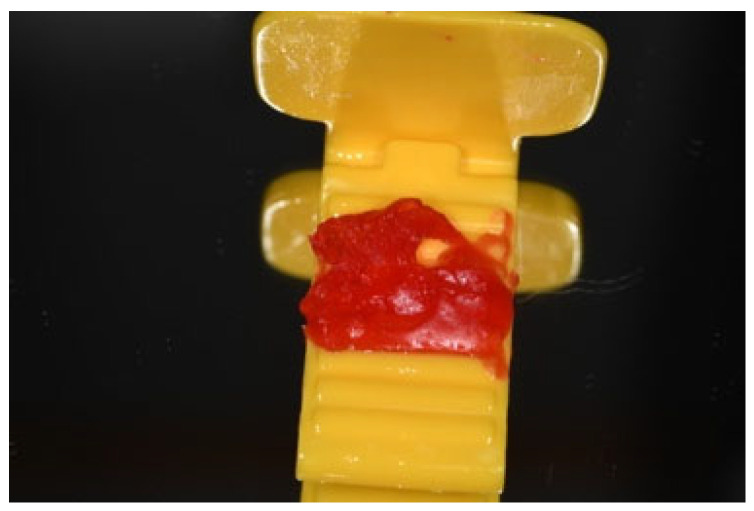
Customized film holder used for each time-point X-ray.
